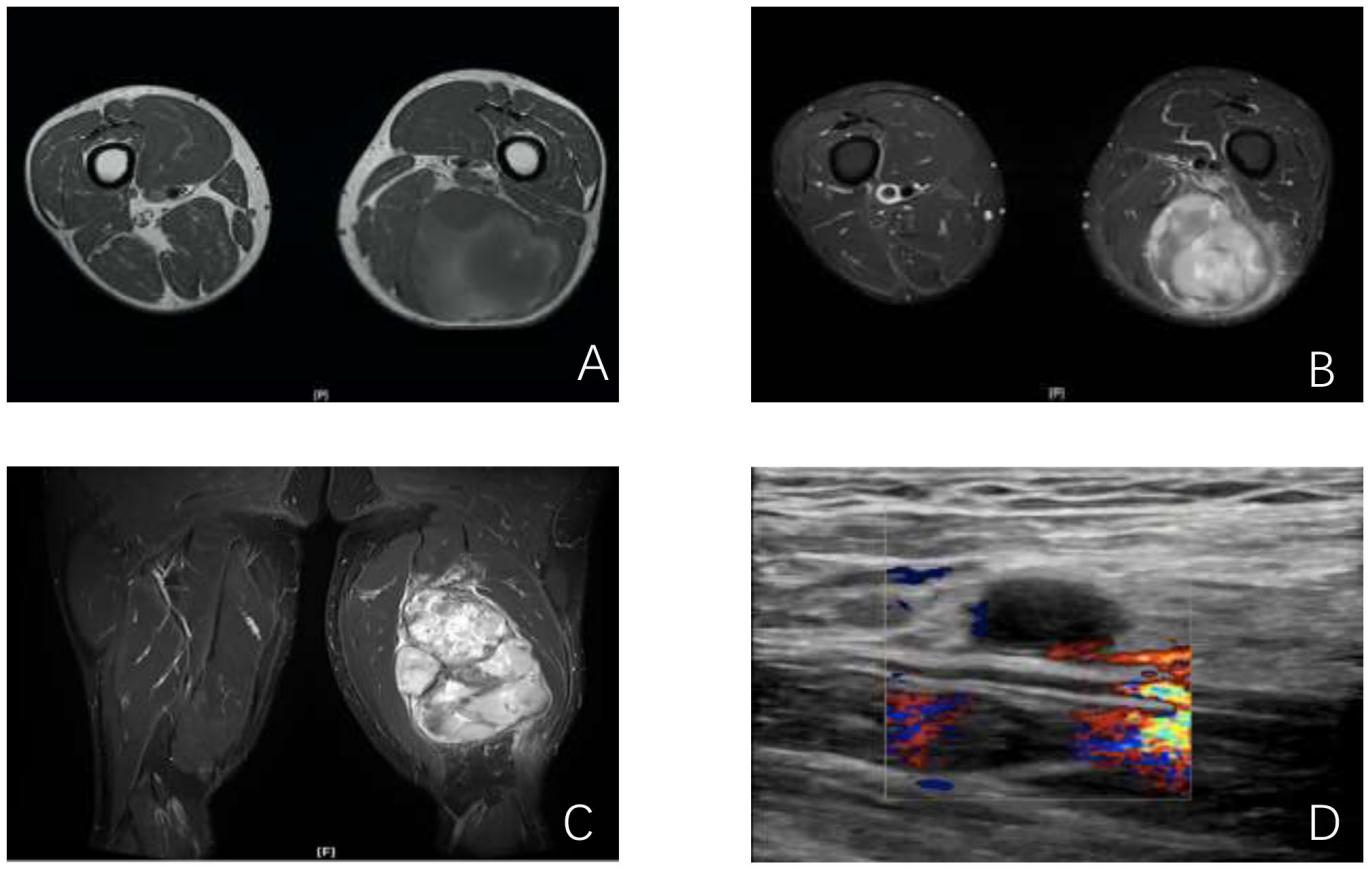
Imaging of the lesions. (A, B) MRI cross sections showing the mass in the left thigh; (C) Lower-extremity arterial computed tomographic angiography (CTA) longitudinal section showing the positional relationship between the mass and the thigh blood vessels; (D) vessel Doppler ultrasonography showing hypoechoic echoes near the left thigh veins.
